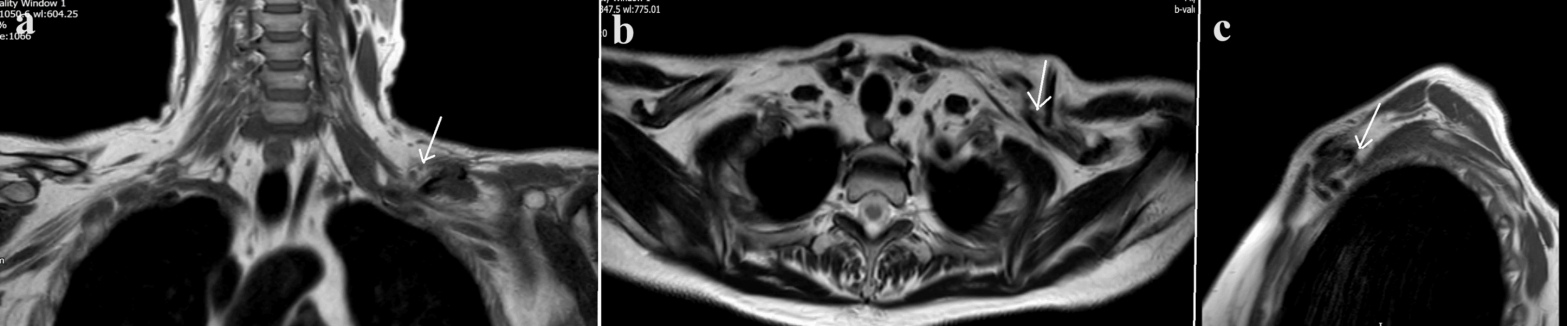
Case 3: Plexus MRI revealing direct compression of the plexus by both the lateral fracture end and the butterfly fragment a, b, and c show the coronal, axial, and coronal views, respectively. The arrow indicates the site of compression.
